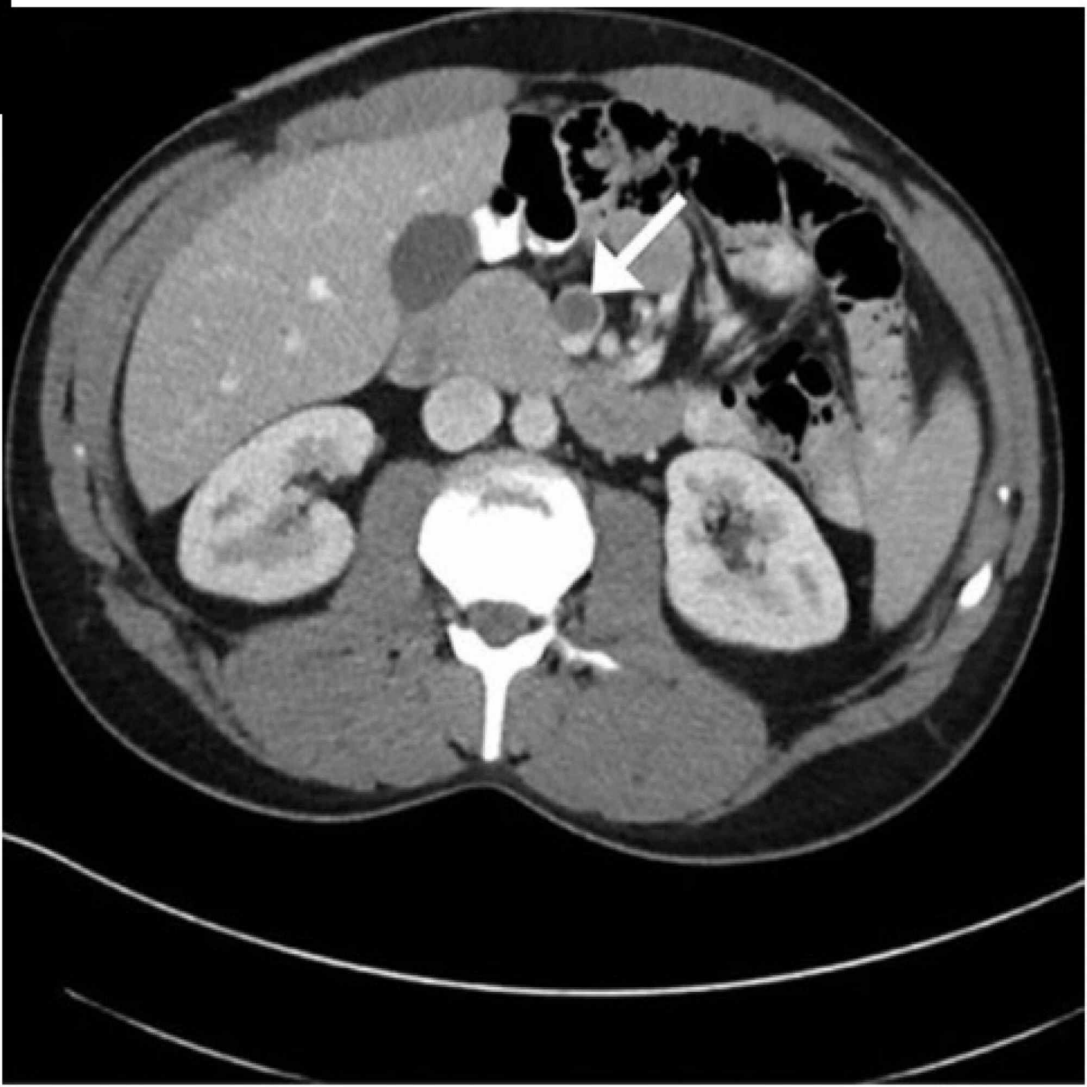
Computed tomography (CT) of the abdomen/pelvis showed pancreatitis with normal biliary ducts and an absence of opacification of the superior mesenteric vein (SMV)
